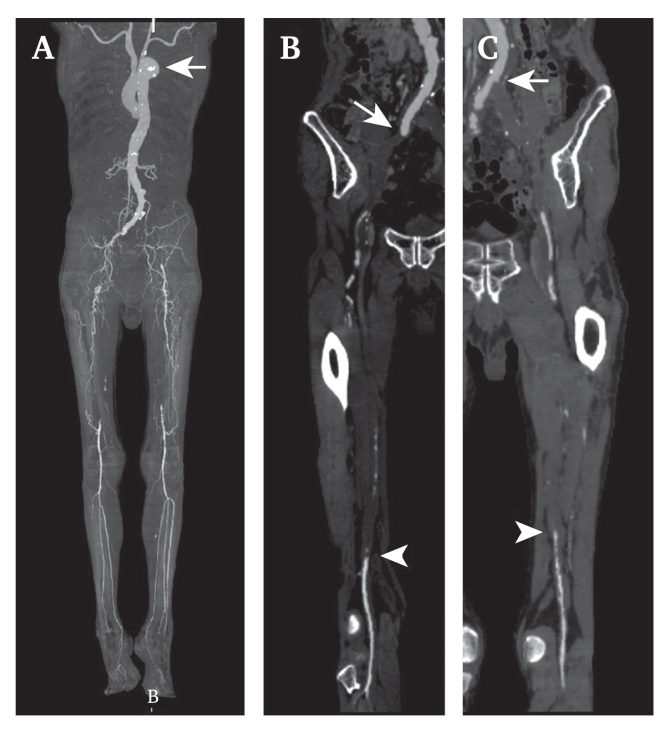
Computed tomography angiography and curved planar reconstruction images. (A) Computed tomography angiography showing long segment arterial occlusions on both sides and thoracic aneurysm formation at the aortic arch (arrow). (B) Curved planar reconstruction image of the artery in the right lower extremity, with the arrow pointing to the start and the arrowhead pointing to the end point of the occlusion. (C) Curved planar reconstruction image of the artery on the left lower extremity, with the arrow pointing to the start and the arrowhead pointing to the end point of the occlusion.
